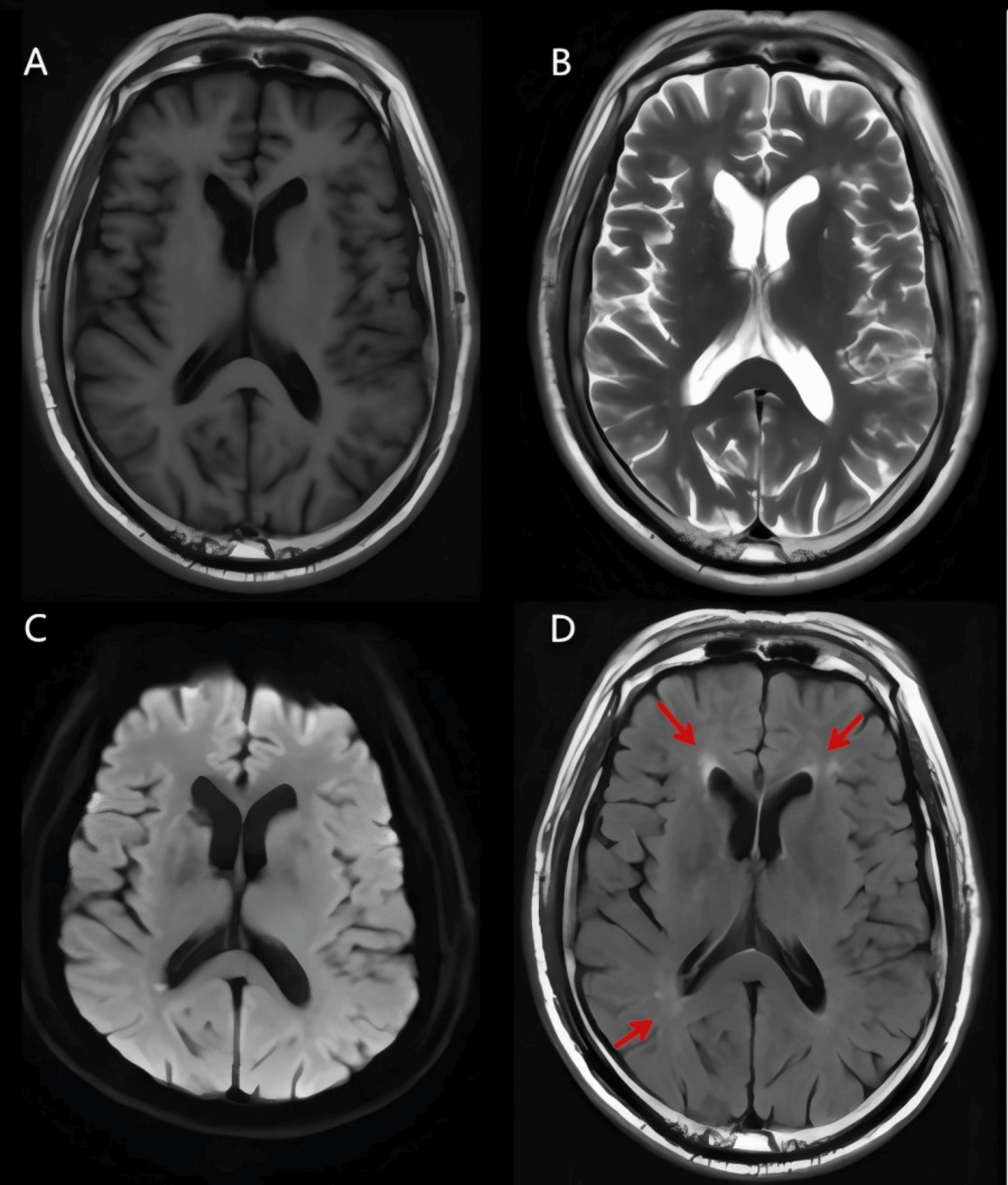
MRI findings of the patient Panels A-C showed no significant structural abnormalities on T1-weighted, T2-weighted, and DWI sequences, whereas panel D clearly demonstrated white matter lesions (marked by red arrows). MRI: magnetic resonance imaging; DWI: diffusion-weighted imaging
